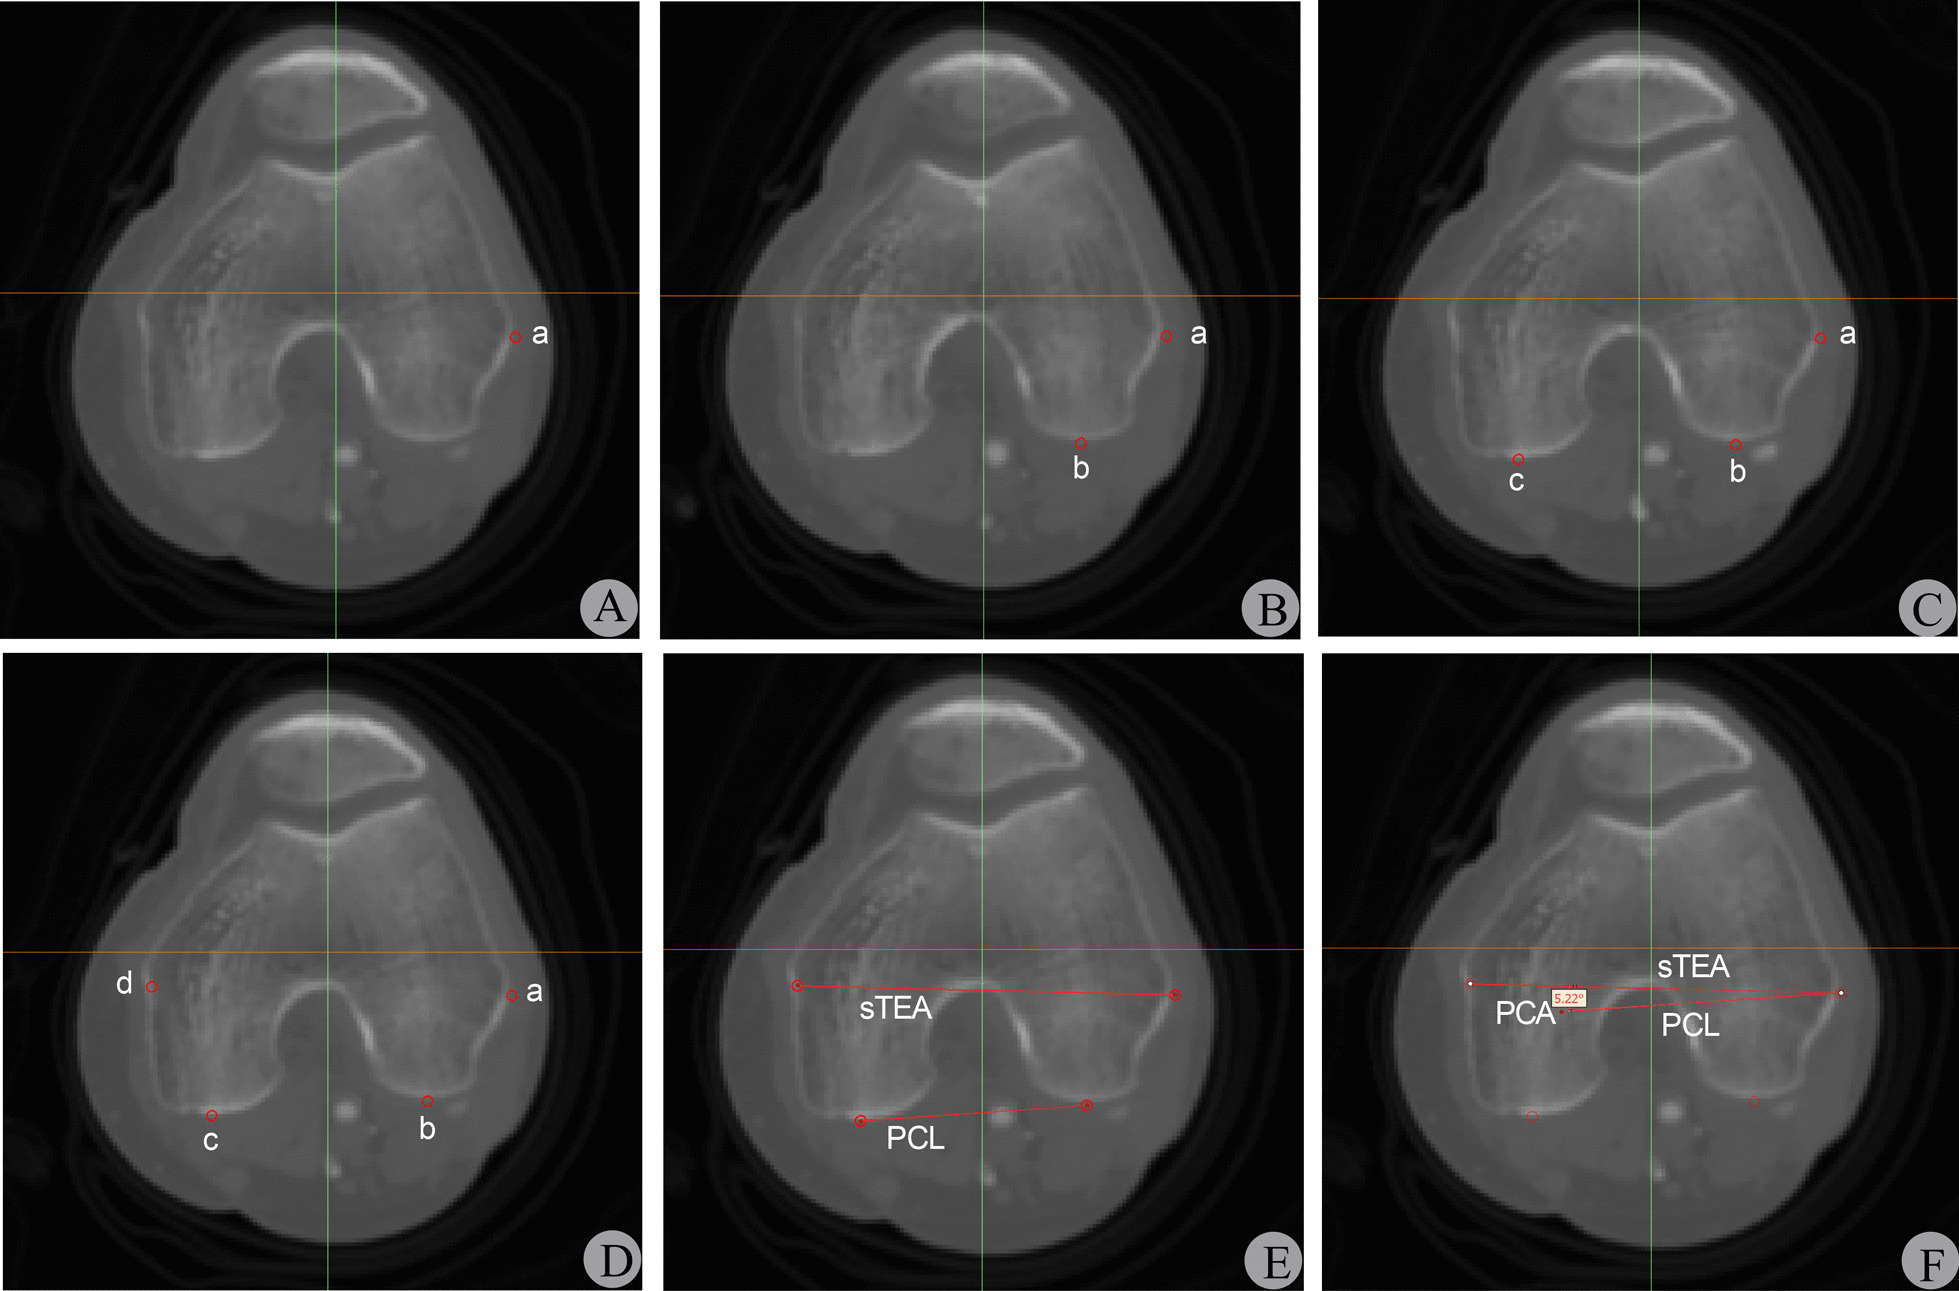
Localization of femoral distal anatomical landmarks and measurement of PCA in multi-plane 2D CT slices. Figure A-D: Localization of femoral distal anatomical landmarks. a-d correspond to the red cylindrical markers placed on the four most obvious points of the most prominent lateral femoral condyle, the lowest points on the lateral and medial posterior condyles, and the medial condyle sulcus, respectively. Figure E–F: Determination of sTEA and PCL and measurement of PCA
